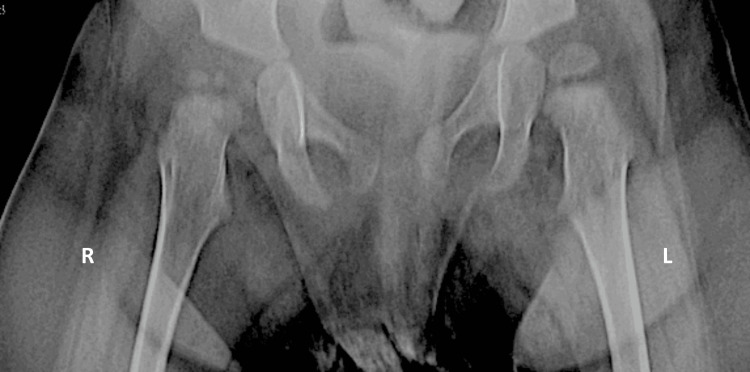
Radiograph obtained prior to discontinuation of the abduction brace showing improved hip positioning but persistent femoral head fragmentation. R: right. L: left
